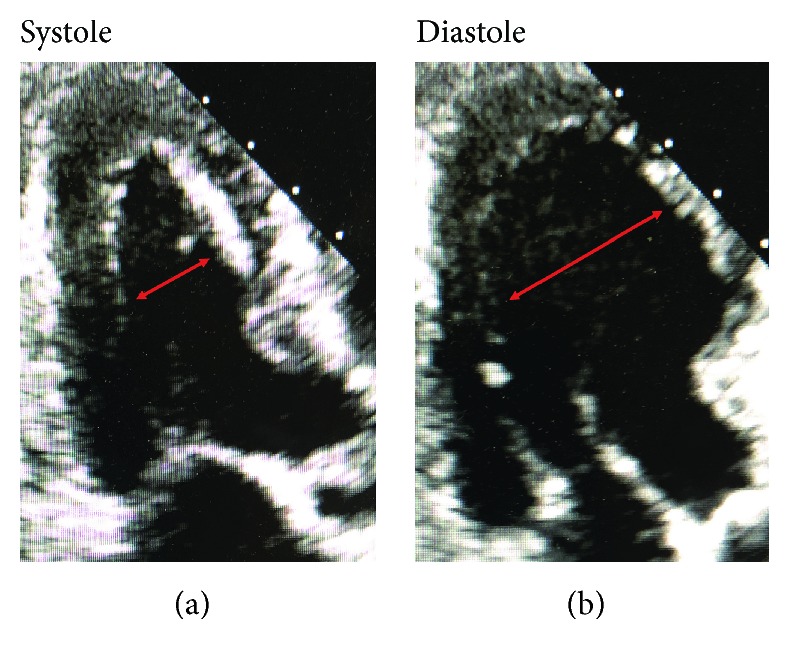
Apical three chamber echocardiogram obtained two weeks following revascularization of the RCA demonstrating no residual left ventricular dysfunction.
